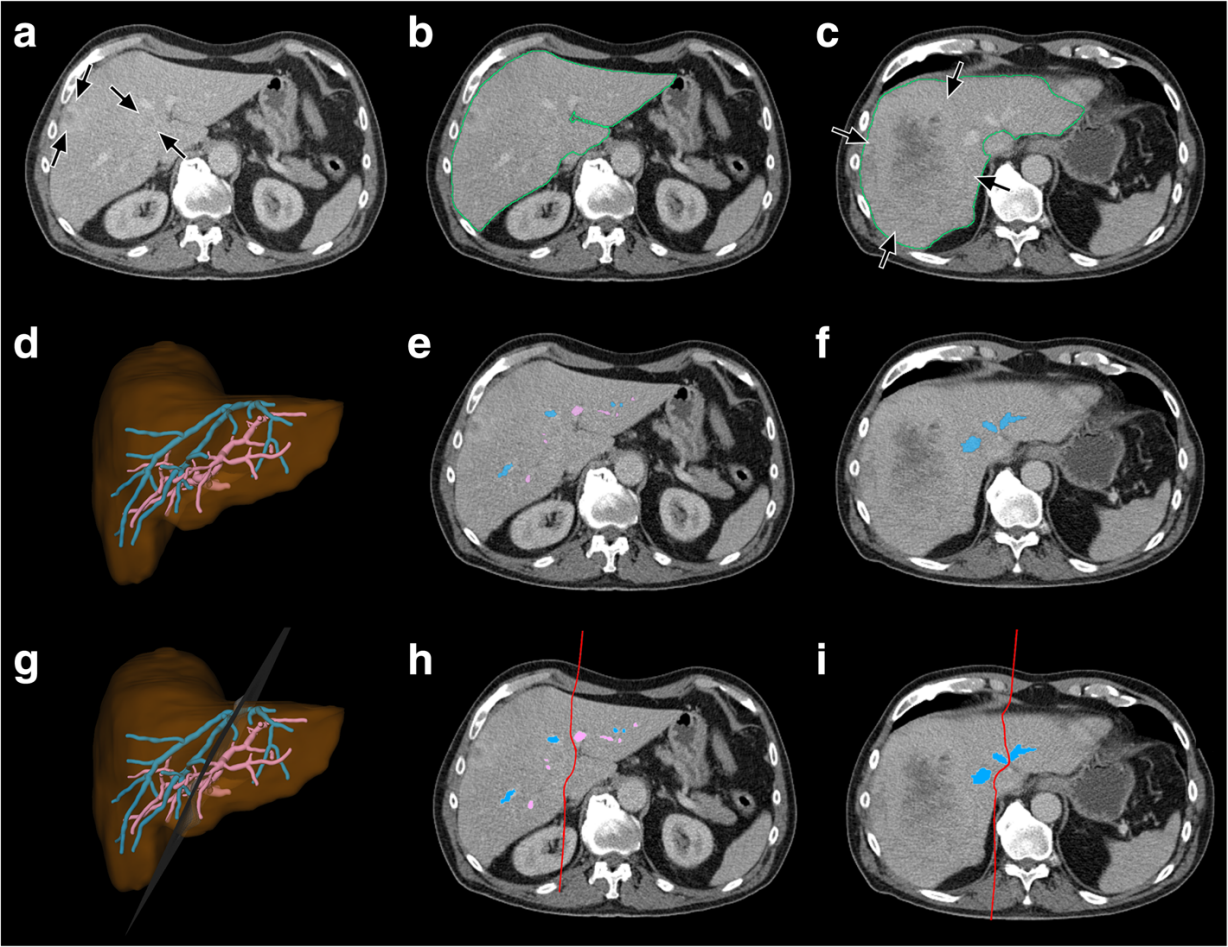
Segmentation process in a patient planned for extended right hemihepatectomy due to metastases of TC a) Plain axial CT image shows small peripherally hyperattenuated metastases (*black arrows*). b) and c) Detection of the liver outline. Another large metastasis is depicted at the level of the proximal MHV in c) (*blue arrows*). d) – f) Detection of the intrahepatic PV (*in blue*) and HV (*in pink*). g) – h) Definition of the transection plane (*in red*)
